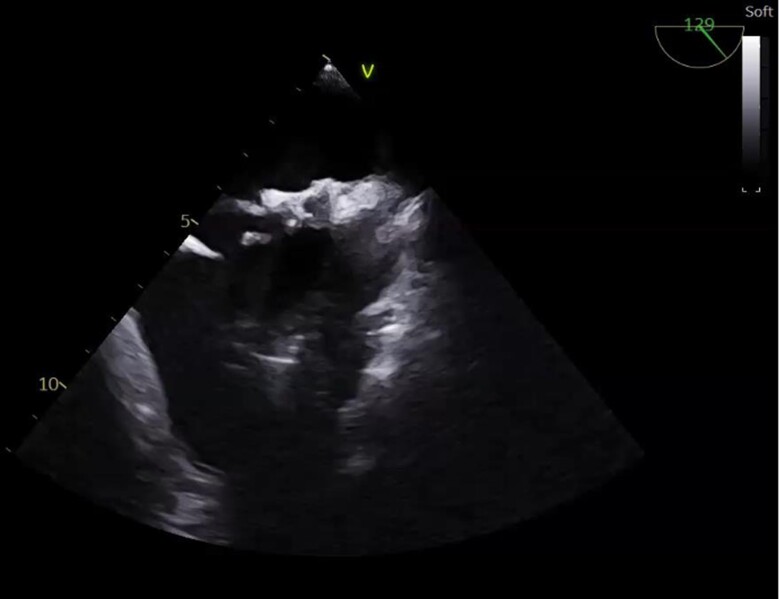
Transthoracic echocardiogram showing the *STARFLEX® prosthesis* with the residual shunt.
